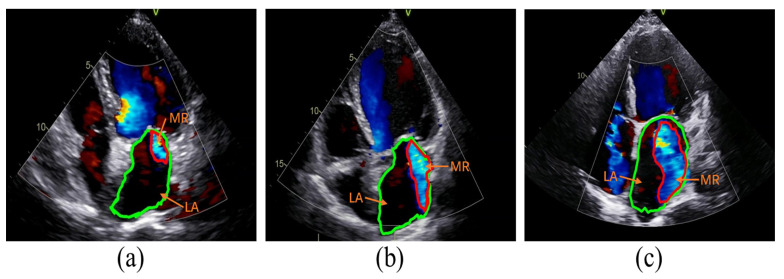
Examples of MR Severity: (a–c) show mild (RF < 30), moderate (30 < RF < 50), and severe (RF > 50) MR, respectively. The images are apical four-chamber views, with the green contour marking the LA border and the red contour marking the MR border. MR: Mitral Regurgitation; RF: Regurgitation Fraction; LA: Left Atrium.
